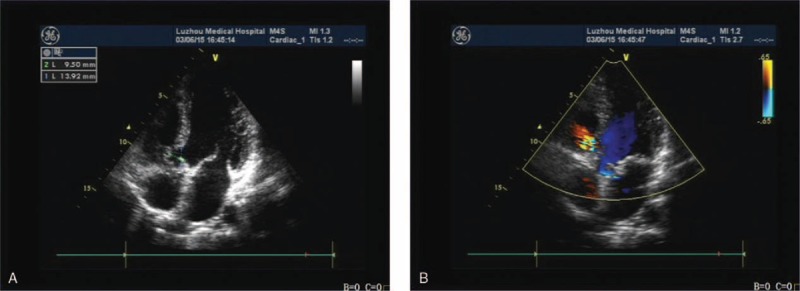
(A, B) Echocardiogram showing evidence of congenital heart disease with a perimembranous VSD. The largest diameter of the defect is 4.9 mm, with left to right shunting at the level of the ventricle.
